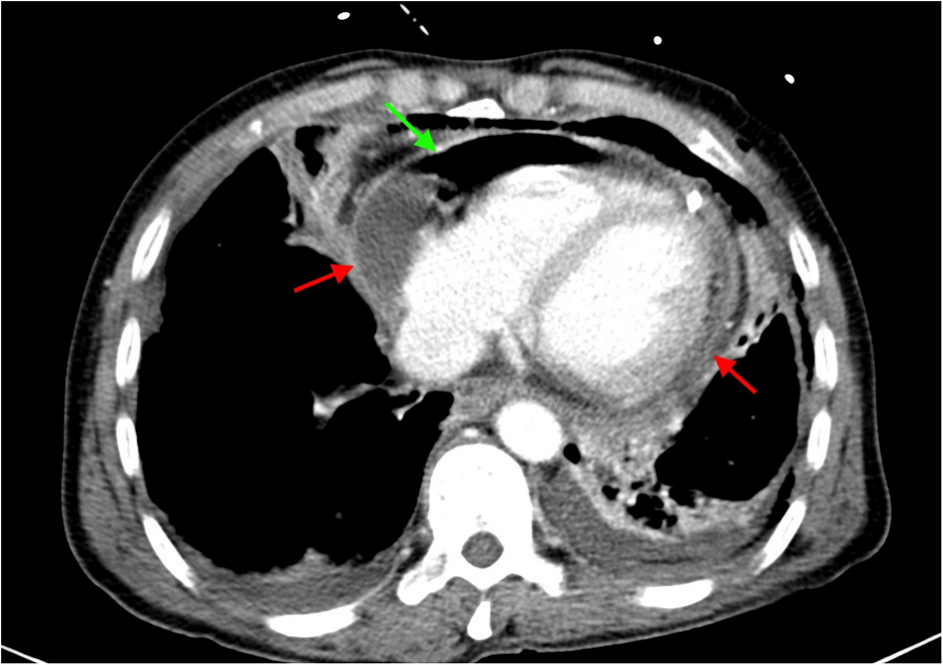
Computed tomography demonstrated enlarged heart with pneumopericardium and effusions in mediastinum and pericardium (green arrow: pneumopericardium; red arrow: effusions).
